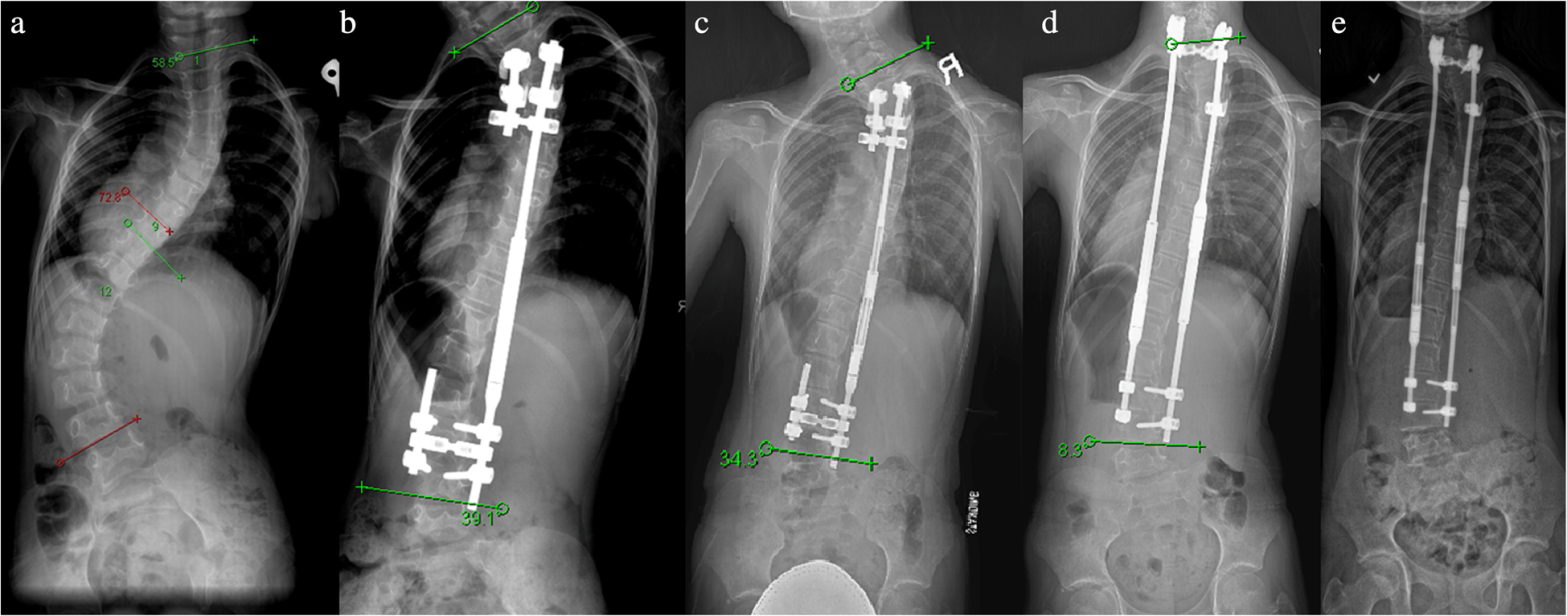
a Preoperative radiograph in 2009 showing 58.5 degrees from T1–9 and 72.8 degrees from T9-L4. b Single MCGR was anchored to T3–4 and L3–4. c The rod was distracted but overall coronal balance was suboptimal. d Dual MCGRs with extension of the proximal foundation to C7-T1 was performed. Note the enlarged portion with the magnet due to the internal keeper plate as compared to the same diameter magnet with the extendable portion of the rod in images b and c. e The rod distracted fully but developed add-on below
